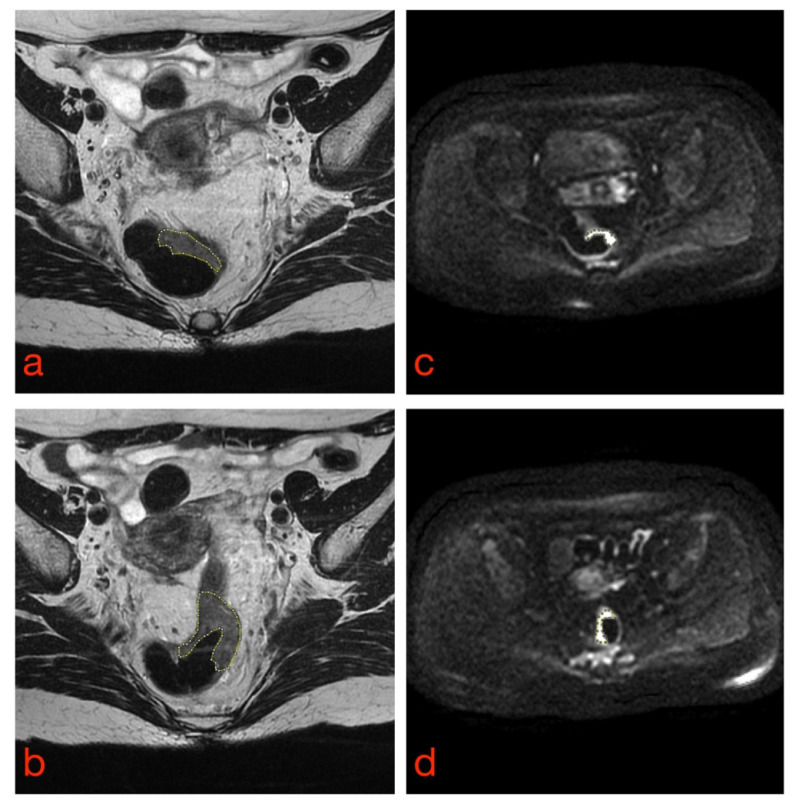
Manual tumor segmentation from T2-weighted (a,b) and DW (c,d) rectal MR images. Tumor borders are highlighted as dashed lines.
